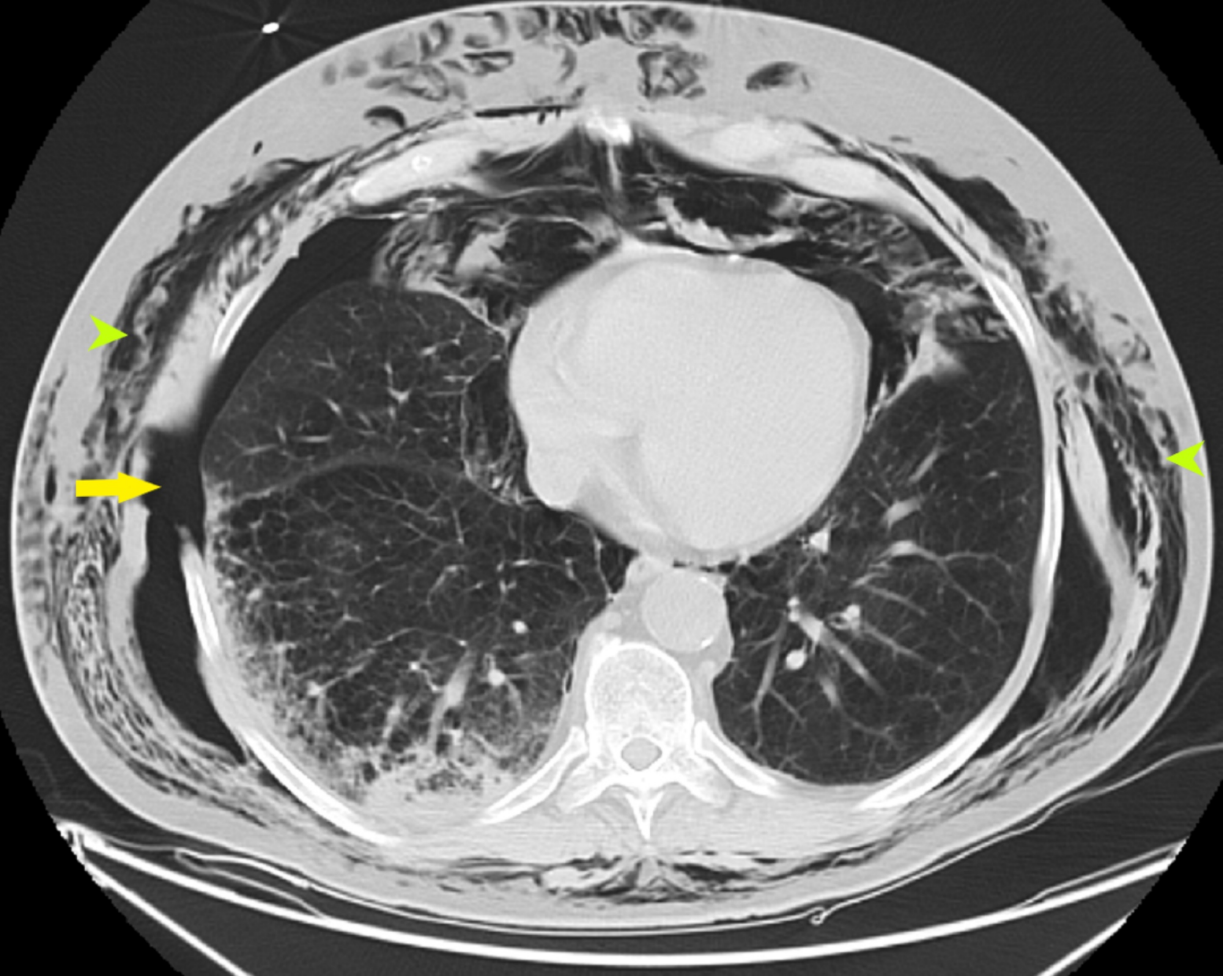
CT chest axial view showing large right-sided pneumothorax (yellow arrow) with extensive subcutaneous air (green arrowheads) throughout the thorax CT: computed tomography
